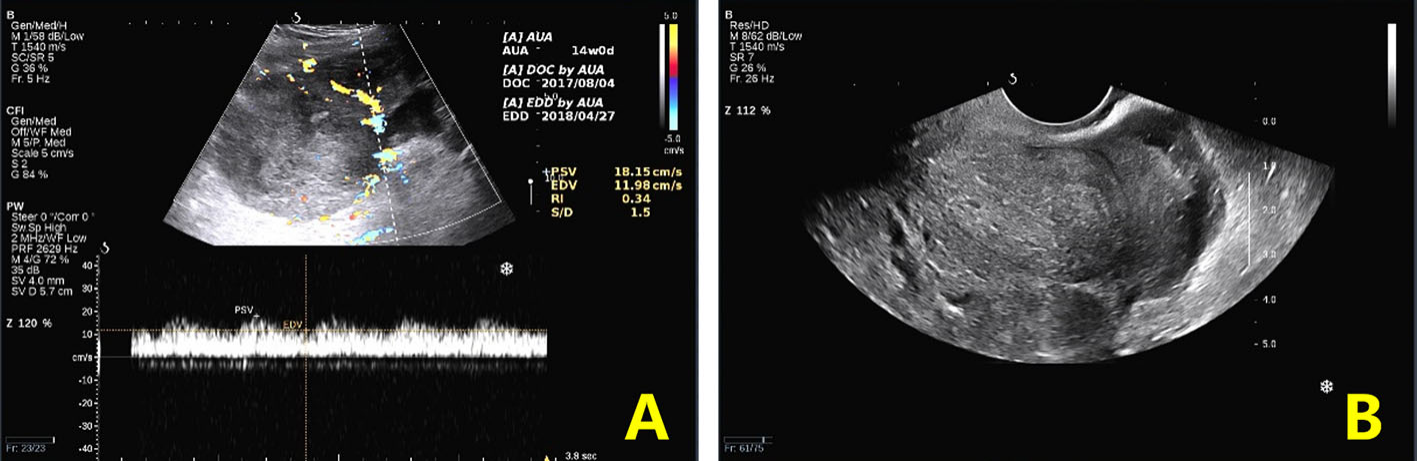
Preoperative ultrasound findings. (A). Transabdominal ultrasound: cross-section view shows a heterogeneous echogenic mass and internal venous and low-resistance arterial blood flow spectrum. (B). Transvaginal ultrasound: longitudinal section of the uterus showing a posterior uterus in the center surrounded by heterogeneous echoes.
